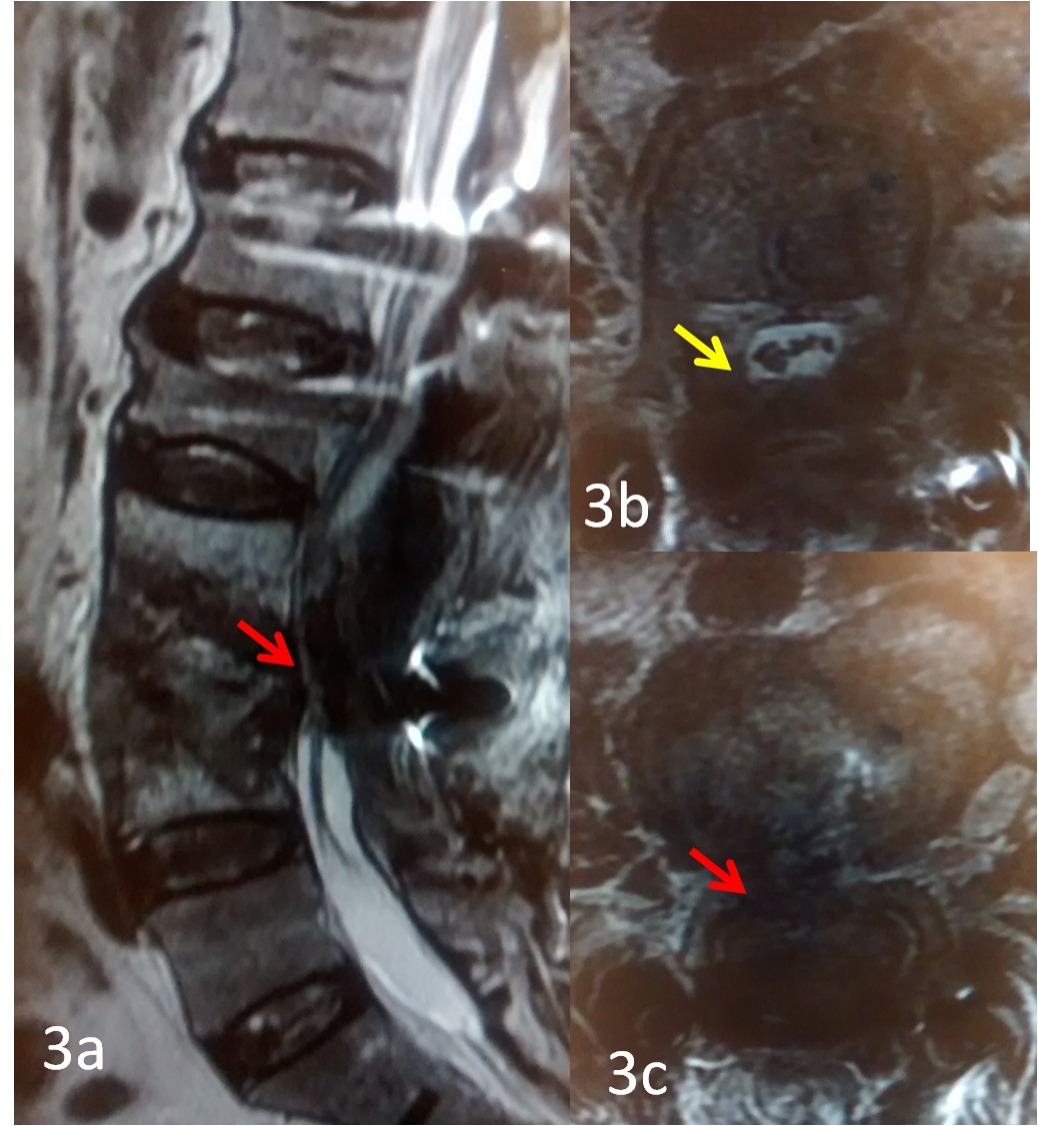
MRI scans of T2W sagittal and axial sections showing marked compression of the thecal sac at L3-4 level Magnetic resonance imaging (MRI) of T2W sagittal showing compression of the thecal sac (3a, red arrow). 3b is the axial image at L2-3, showing no thecal sac compression, for comparison (yellow arrow). 3c shows the axial image at L3-4 with the complete occlusion of the thecal sac (red arrow).
